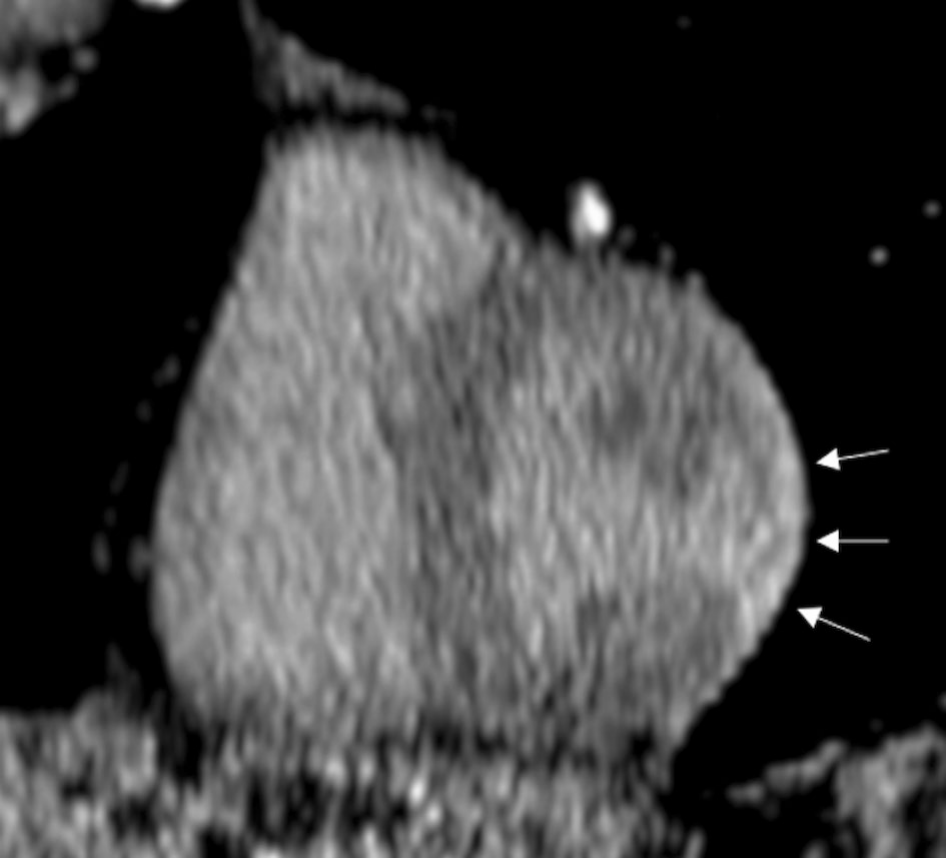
CT examination performed in a patient with chest pain. Late phase acquisition (8 min after contrast administration) using low Kv. Short-axis reformation shows subepicardial enhancement in the mid-ventricular lateral wall, suggestive of myocarditis. The diagnosis was later confirmed by lab test and MRI scan
